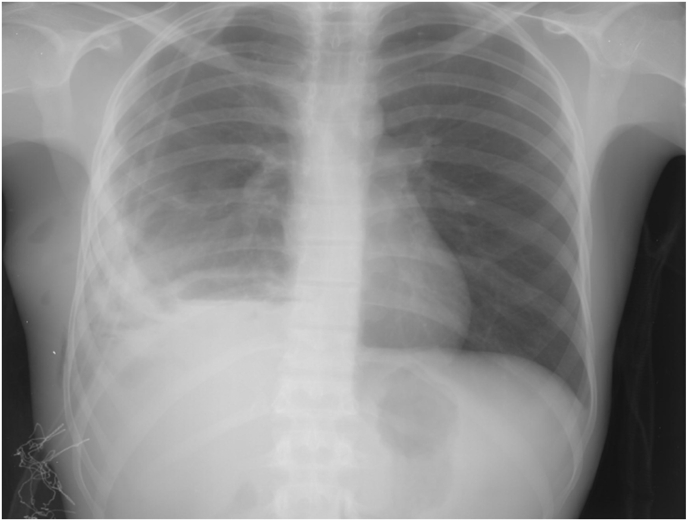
Patient's chest X-ray 3 days postoperatively, a fully re-expanded right lung.
